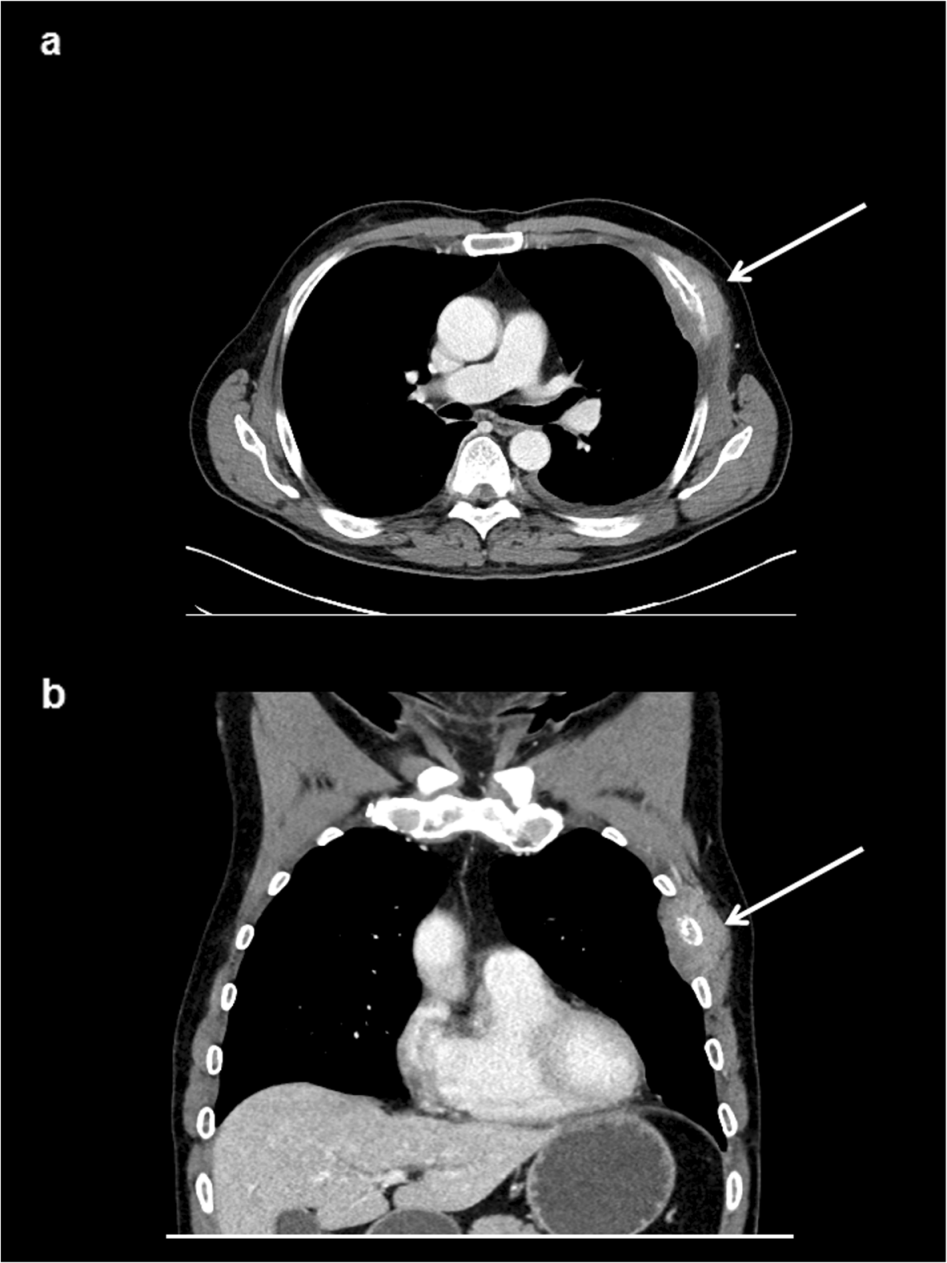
Myeloid sarcoma of the chest wall in a male patient with known acute myeloid leukemia in a relapse setting. Contrast enhanced computed tomography in axial (a) and coronal plane (b) showing a relatively homogenous of the third left rip with an infiltration of the adjacent intercostal muscles (arrows)
